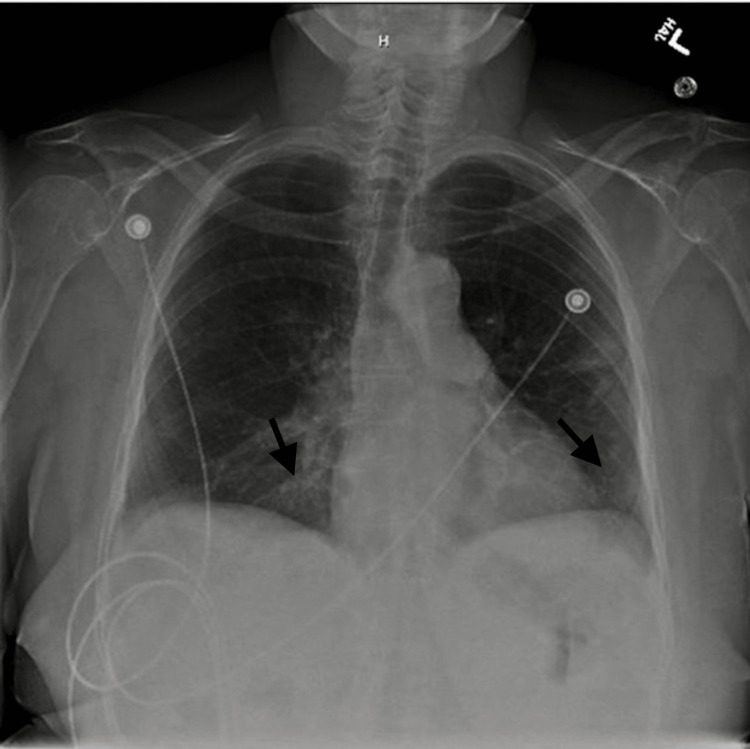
A chest radiograph showing mild infiltrates in the left lower lung and right lung base (arrow)
